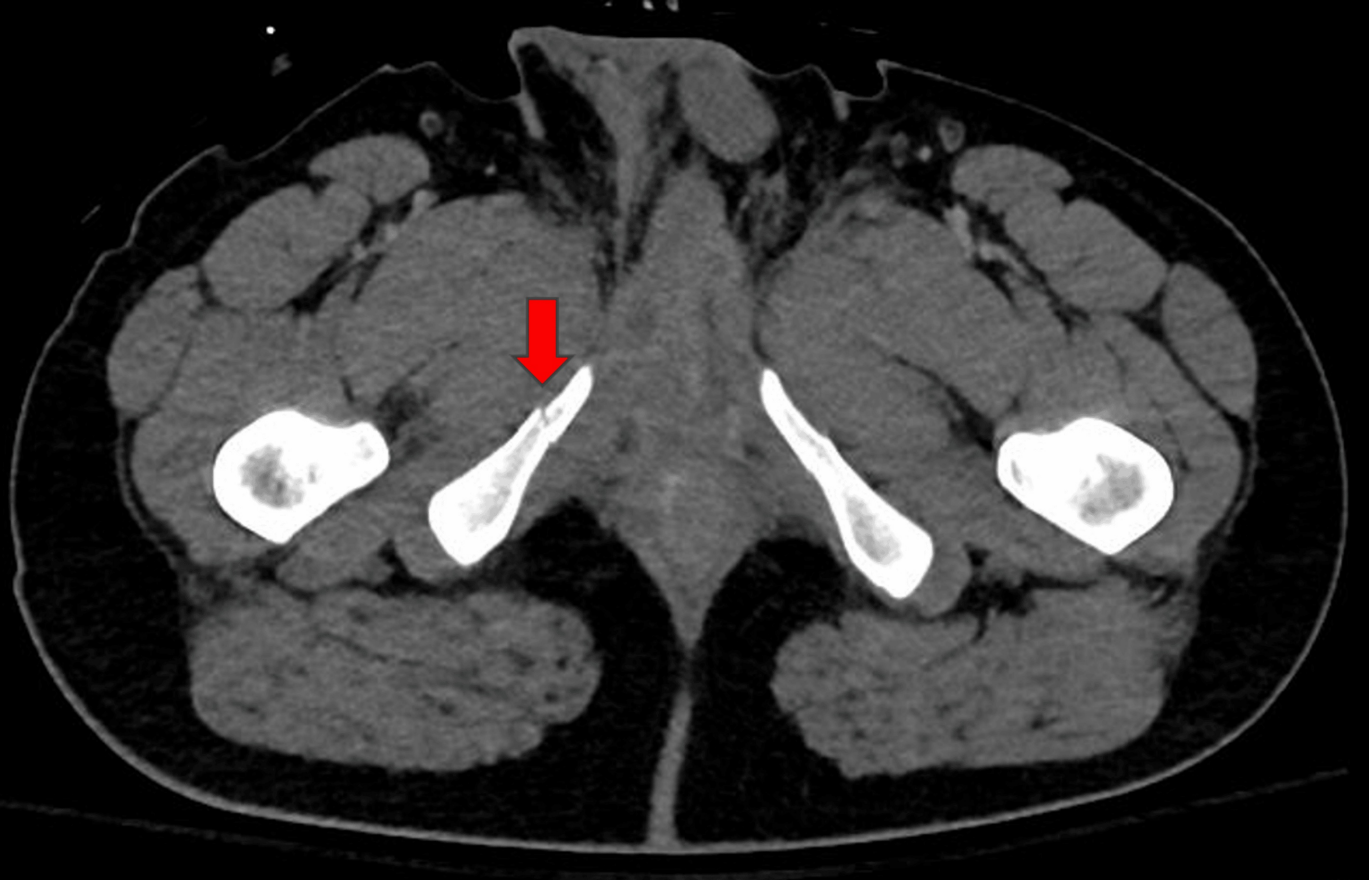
CT abdomen/pelvis demonstrating nondisplaced pubic fracture (red arrow). CT: computed tomography
